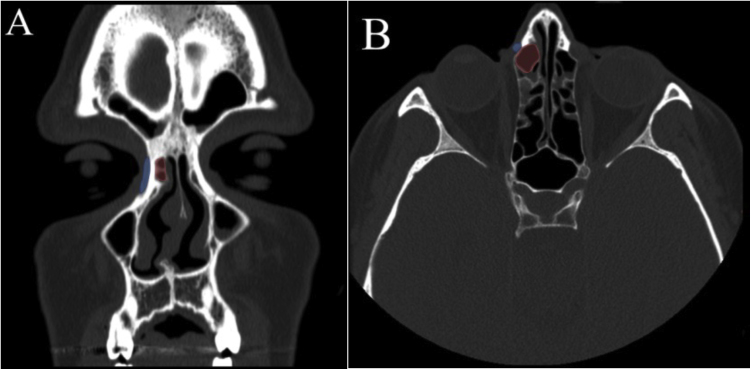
Agger nasi cell located medial to the lacrimal sac. (A) CT scan (coronal slice) showing agger nasi cell (red) medial to the lacrimal sac (blue) at the level of the common canaliculus on the right. (B) CT scan (axial slice) showing agger nasi cell medial to the lacrimal sac on the right.
